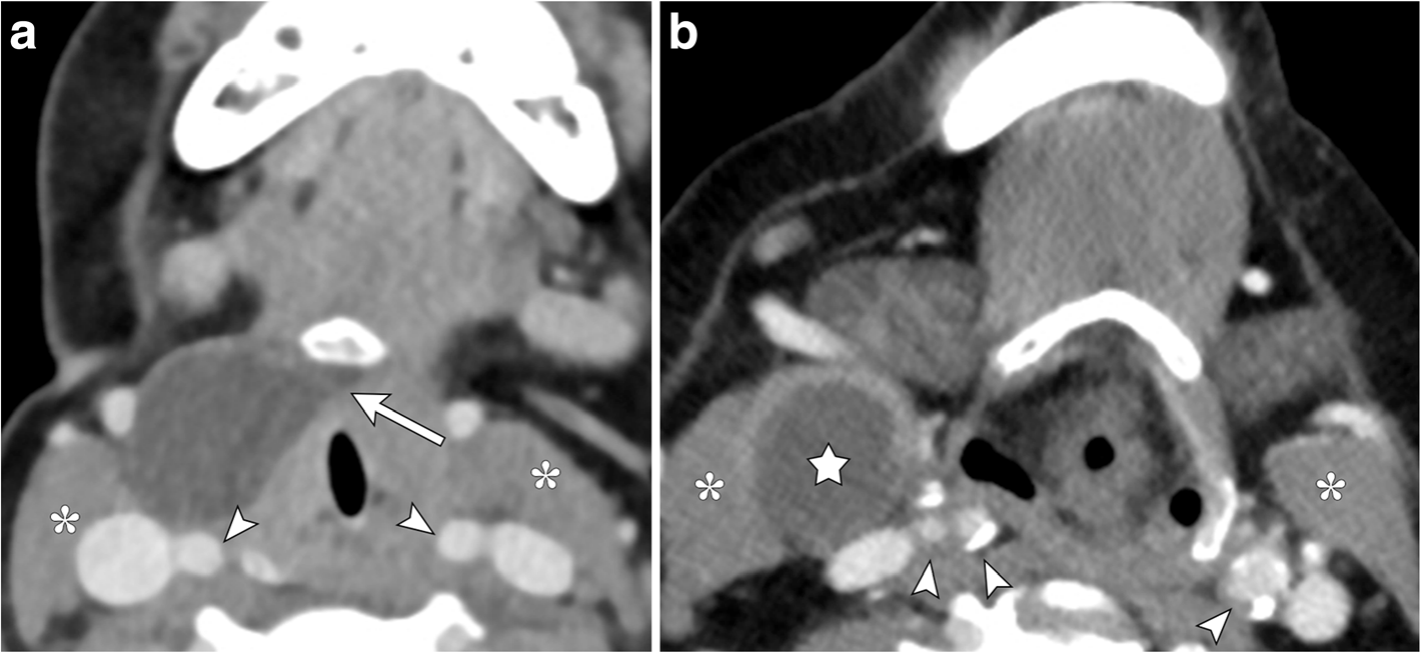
Thyroglossal duct cyst and branchial cleft cyst. a Axial contrast-enhanced CT image shows a pathology proven thyroglossal duct cyst located anterior to the carotid vessels (arrowheads) and anteromedially to the sternocleidomastoid muscle (asterisks) with a tail-like extension medially to the hyoid bone (arrow). b Axial contrast-enhanced CT image demonstrates a second branchial cleft cyst (star) in a similar location within the right neck without hyoid extension
